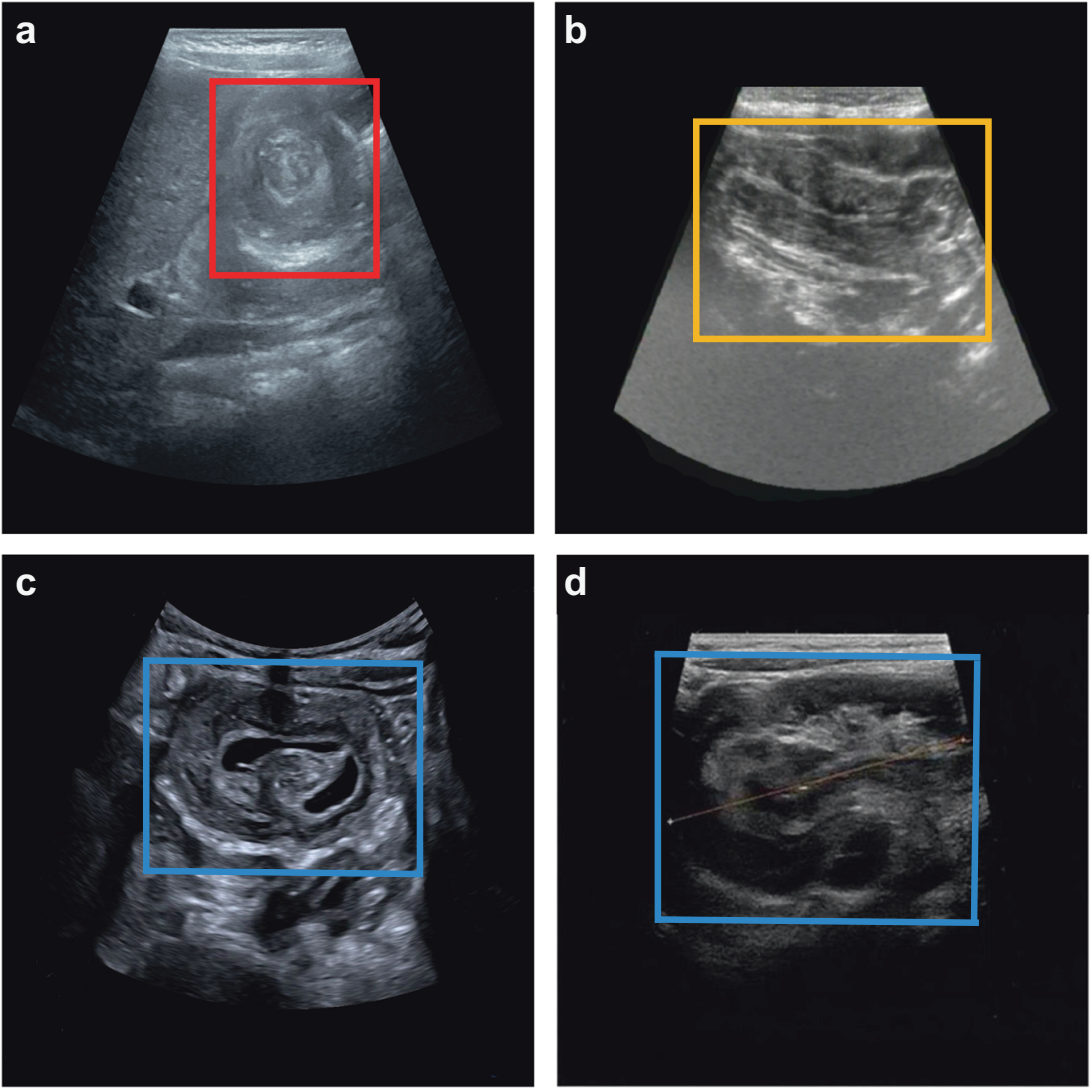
Three types of ileocolic intussusception lesions were labeled by ultrasound experts. a NSD Nonsurgical doughnut sign. b NSS Nonsurgical sleeve sign. c, d SSI Surgical sign.
